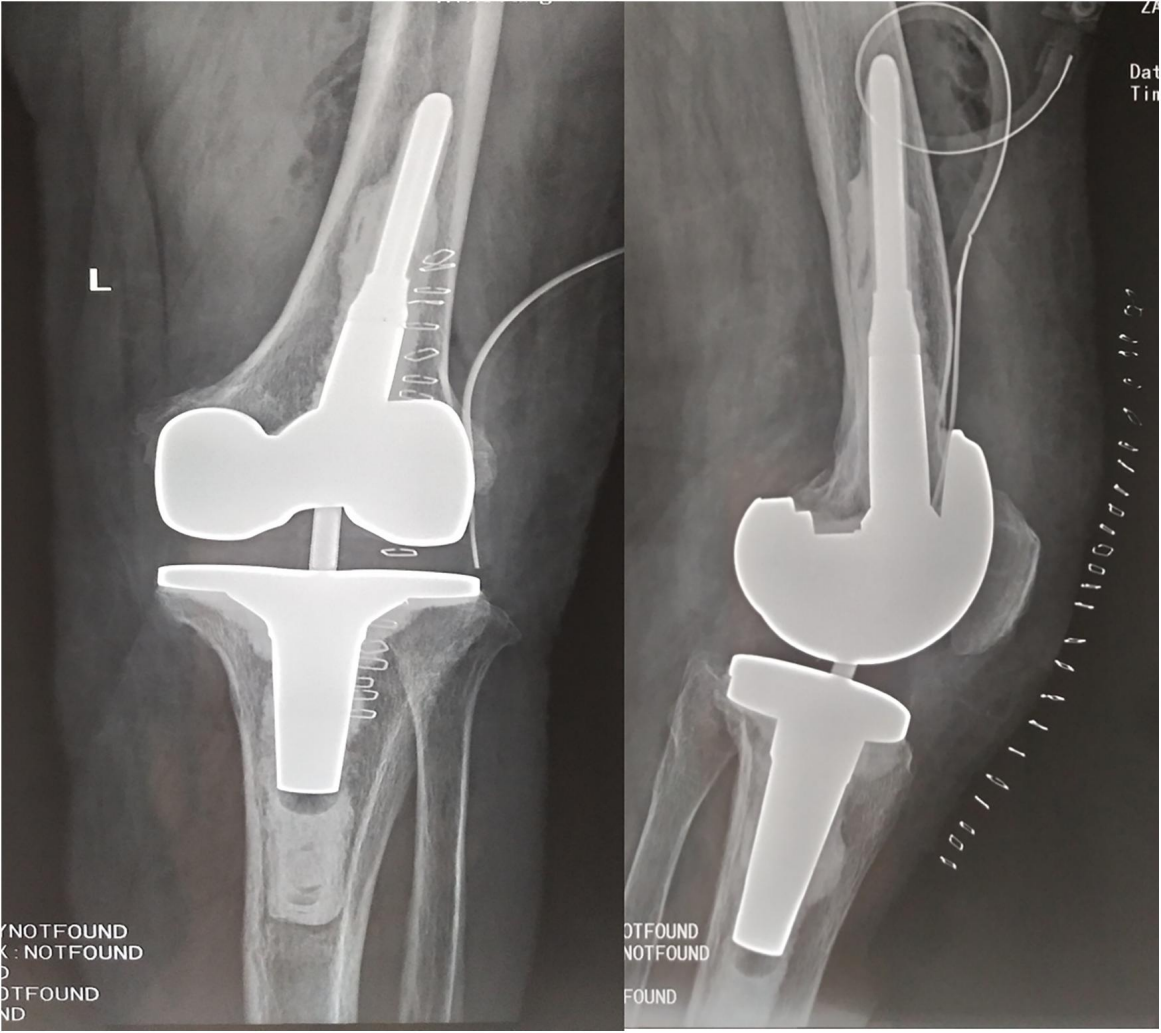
Post-operation x-ray shows no prothesis loosening happened, implants placed good position.
